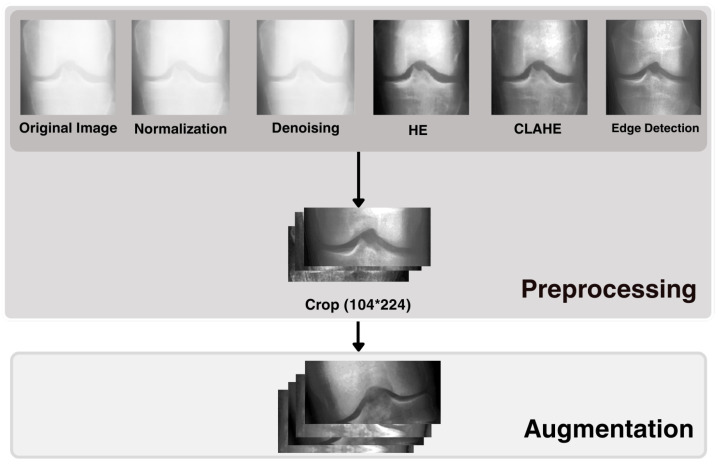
Preprocessing operations applied to knee X-ray images.
